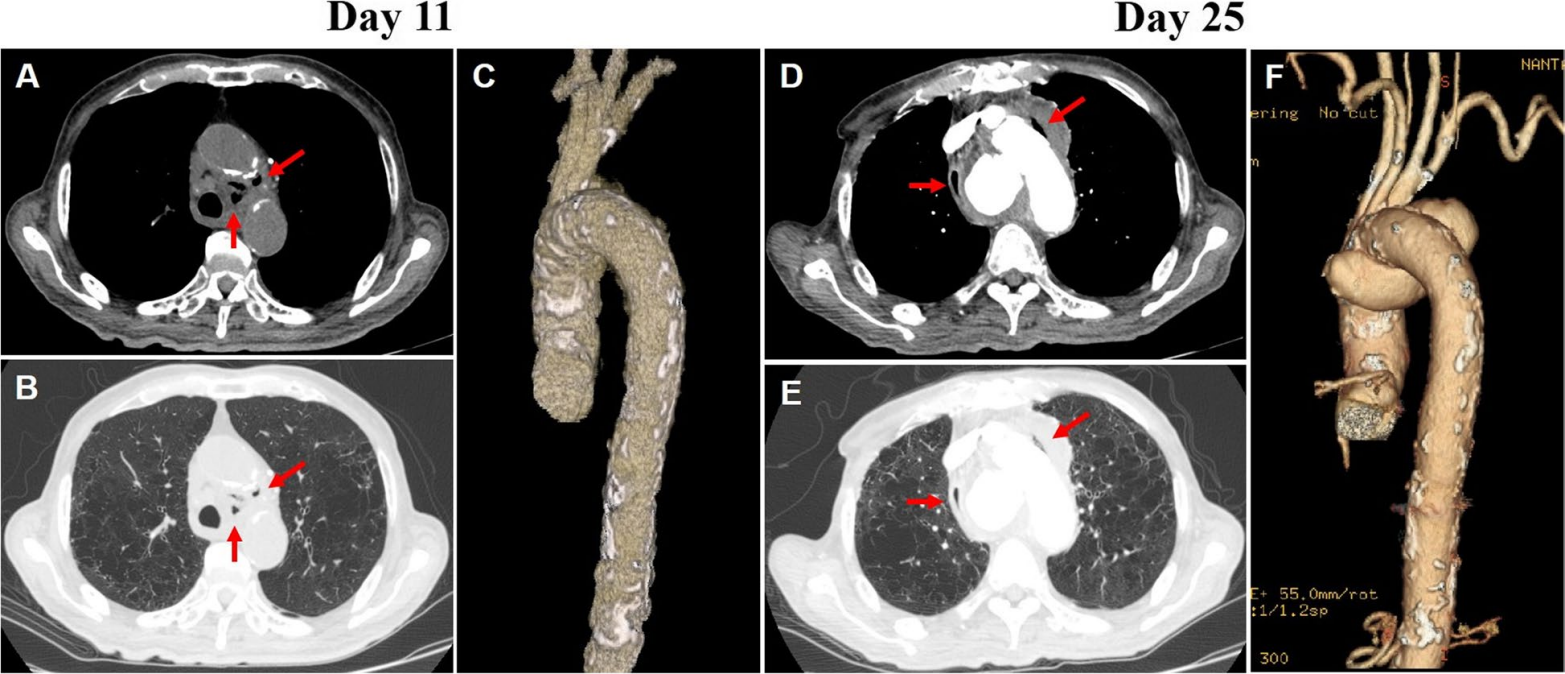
a, b A chest computed tomography (CT) showing mediastinal emphysema (Arrows). c The thoracic aorta exhibited some atheromatous changes but no aneurysmal formation. d, e A contrast-enhanced chest CT showed peri-aortic emphysema (Arrows). f Three-dimensional CT angiography demonstrated marked pseudo aneurysmal formation around the aortic arch
